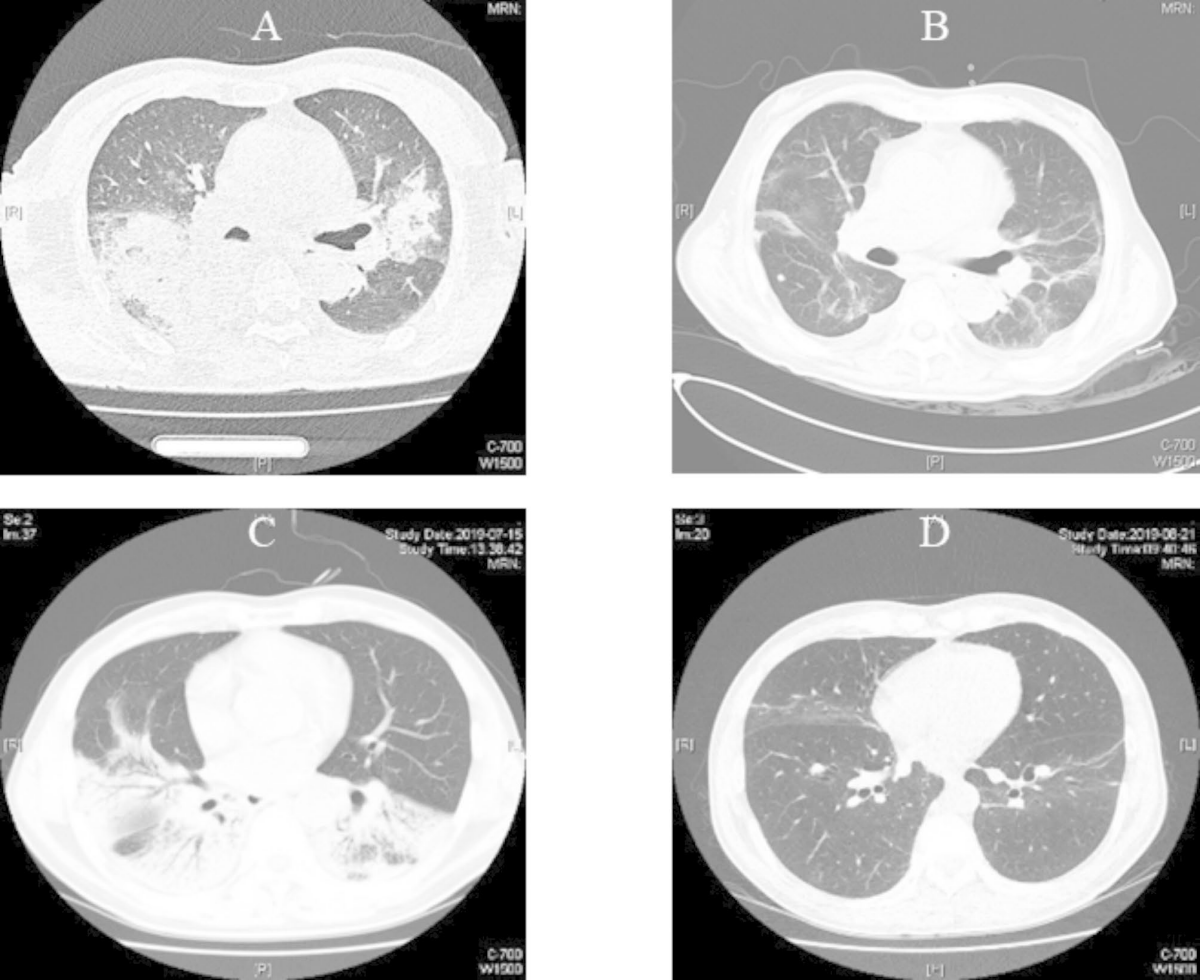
Lung computed tomography findings of the patients with psittacosis caused severe community acquired pneumonia Figure-2A was the lung CT of an 80 years old male of psittacosis pneumonia while admission, which showed consolidation and infiltrate in bilateral lungs. Figure-2B showed reticular lesions and ground-glass opacities residually in bilateral lungs before discharged from ICU. Figure-2C showed a 54 years old female, consolidation and infiltrate in lower lobes of bilateral lungs. Figure-2D showed few reticular in bilateral lungs before discharged
